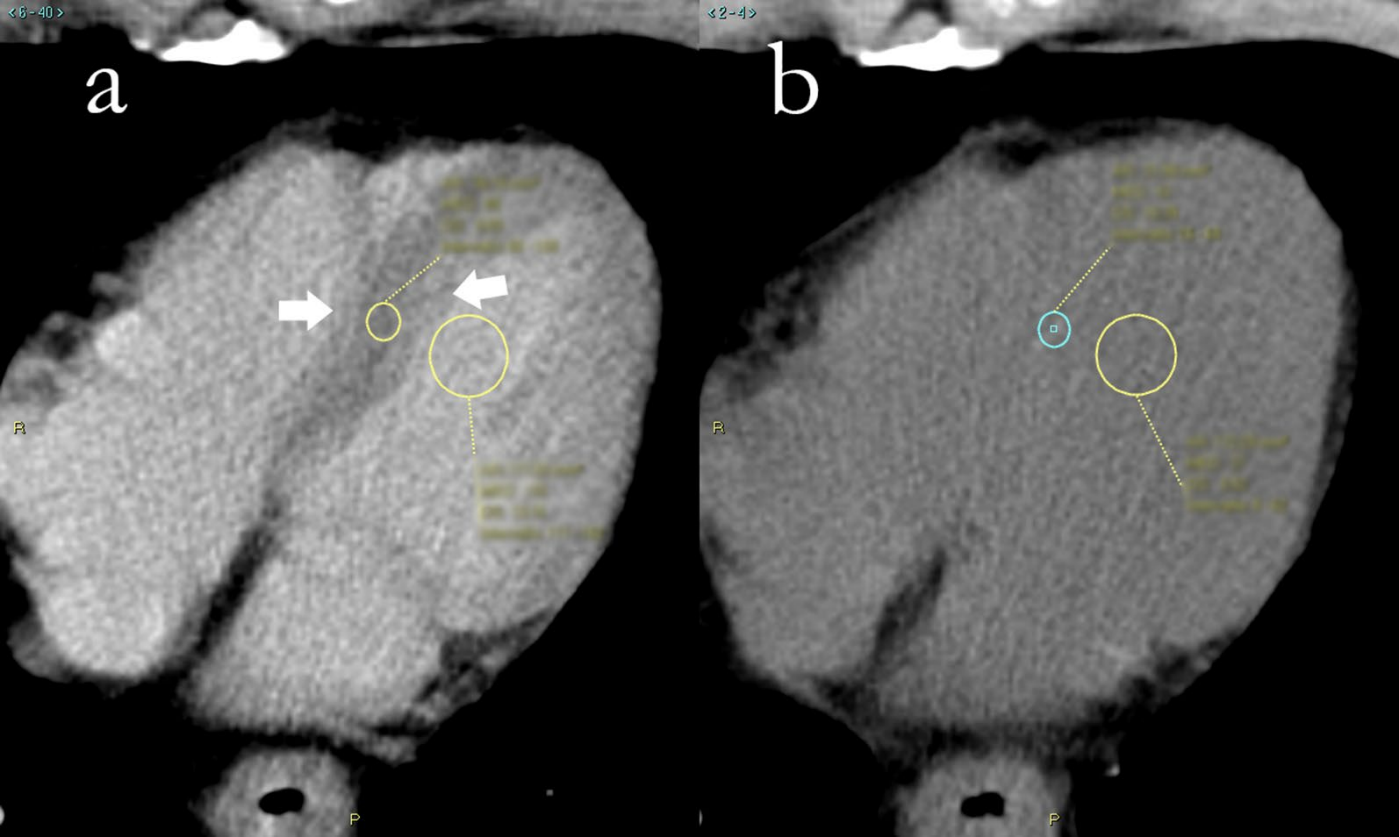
Region of interest placement for extracellular volume calculation in computed tomography of a 65-year-old male patient with adenocarcinoma of lower esophagus. a Contrast-enhanced scan, where papillary muscles are visible and thus avoided. The septum is clearly distinguishable from the intraventricular blood pool, and a certain degree of blurring is noticeable toward the borders (white arrows). b Unenhanced scan. Myocardial tissue is almost unrecognizable from the intraventricular blood pool, and regions of interest are placed in roughly the same position of a and then adjusted following local attenuation measurement
